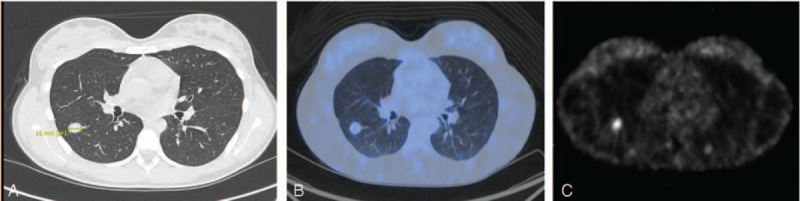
(A) The broncopulmonary nodule detected (diameter 16 mm) was located at the apical segment of of the right lower lobe (RLL). The nodule showed (B) a SUVmax of 3.5 at ^18^FDG-PET/CT and (C) a SUVmax of 3.4 at ^68^Ga-PET/CT. SUV = standardized uptake value.
